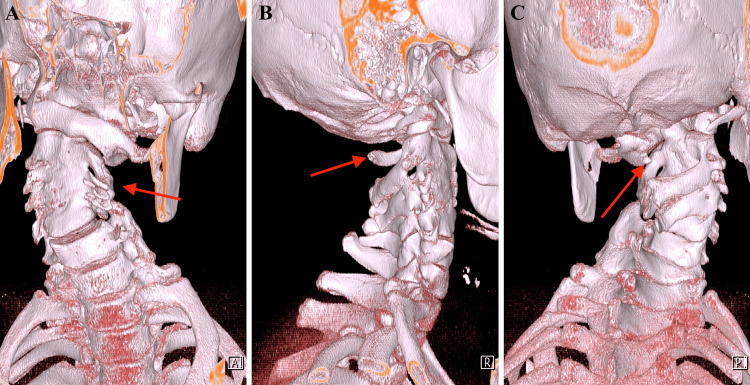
3D reconstruction of the CT scan CT, computed tomography; 3D, three-dimensional (A) Cervical fusion from C2 to C5 (red arrow). (B,C) Anomaly of the C1 vertebral arch (red arrow).
